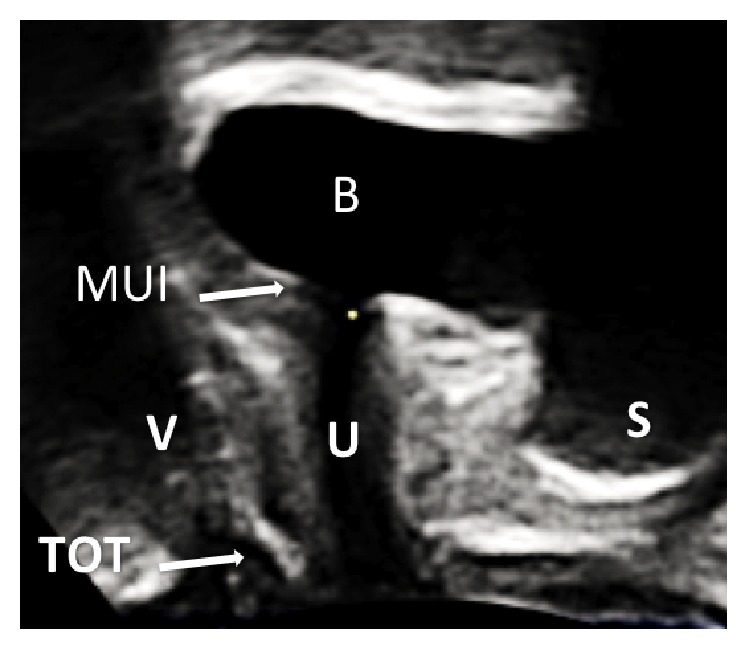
Perineal ultrasound image on midsagittal plane from a patient at rest with a tension-free transobturator tape (TOT). The positions of symphysis pubis (S), bladder (B), meatus urethrae internus (MUI), urethra (U), and vagina (V) are indicated.
